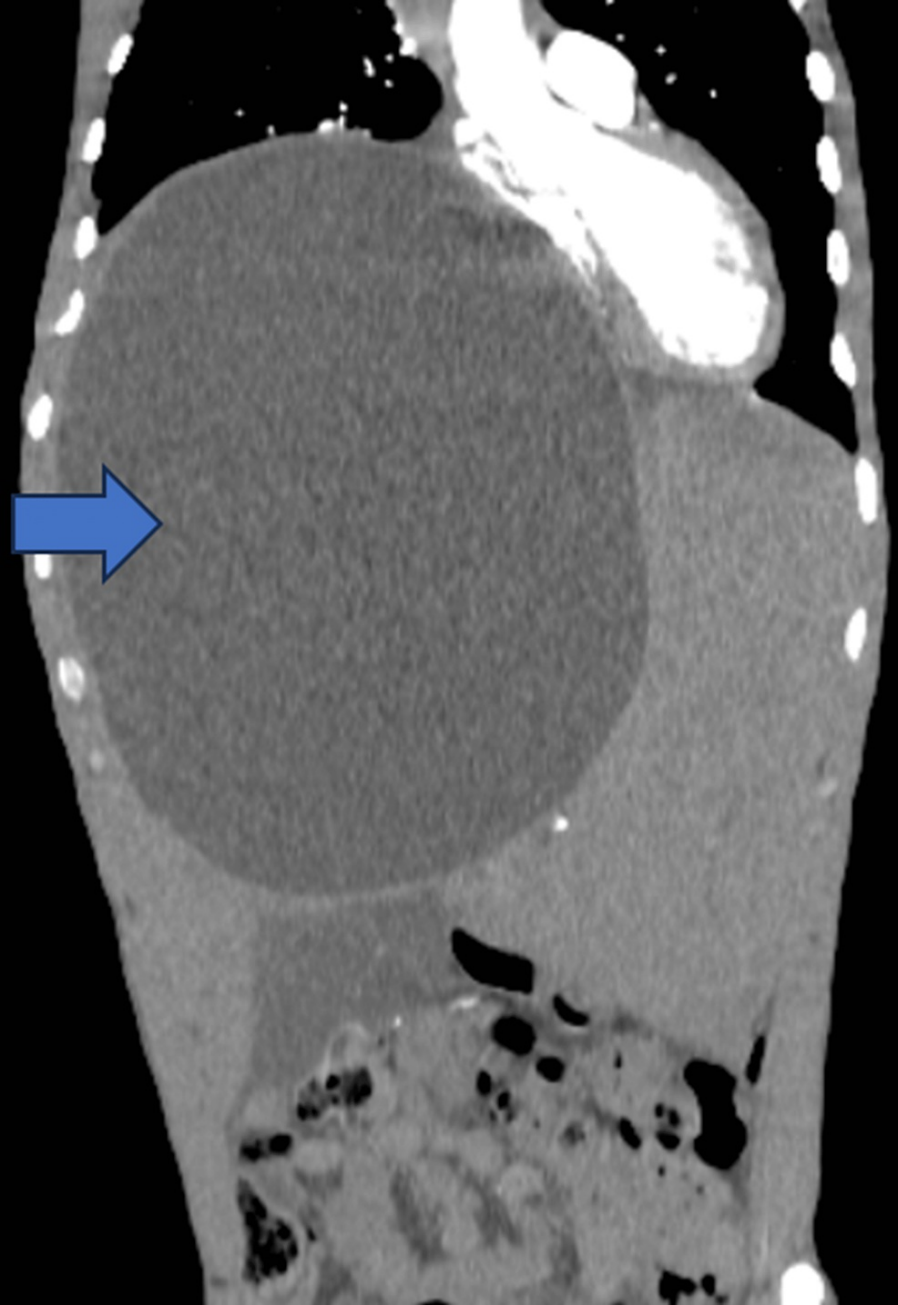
Coronal view of an abdominal CT image showing the cyst (blue arrow) displacing mediastinal structures to the left. CT: computed tomography.
